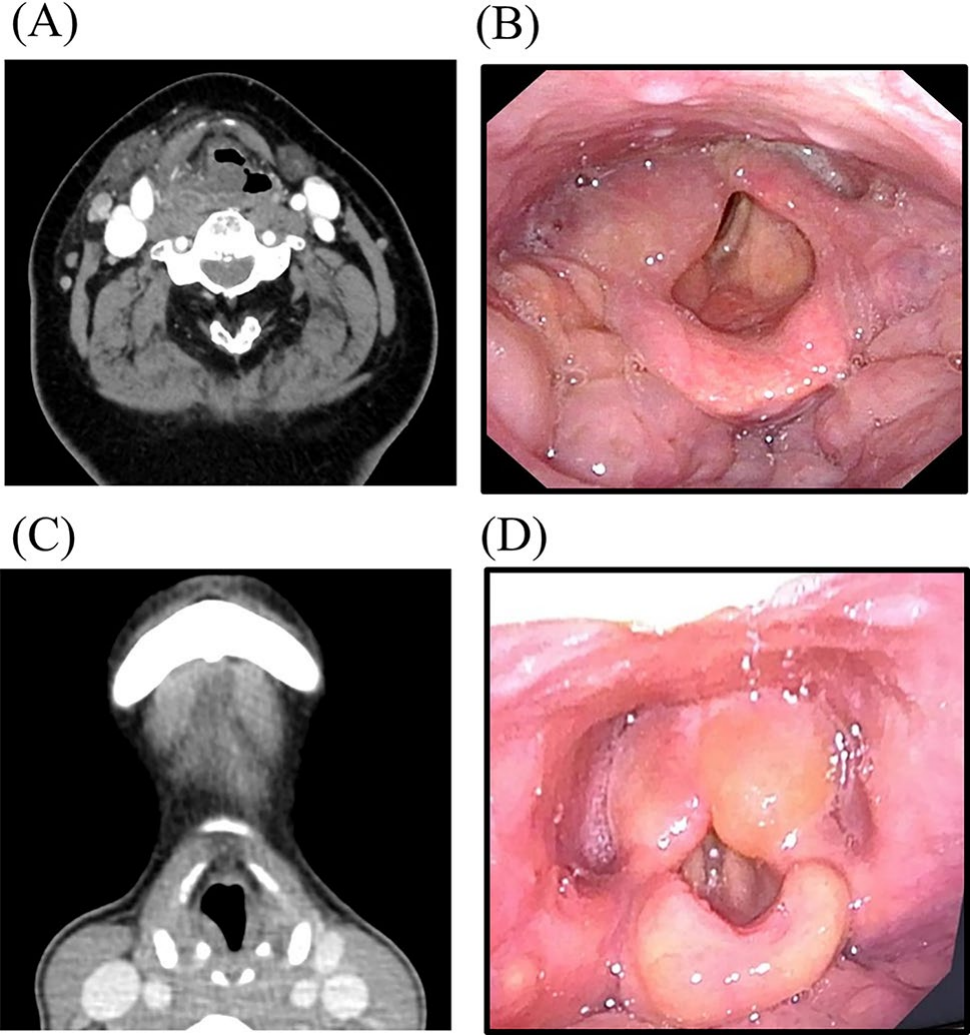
Grading of laryngeal edema (epiglottis swelling). Grade I: swelling on one side of the axial CT image (A) and laryngoscopy image (B). Grade II: swelling on both sides on axial CT image (C) and laryngoscopy image (D)
